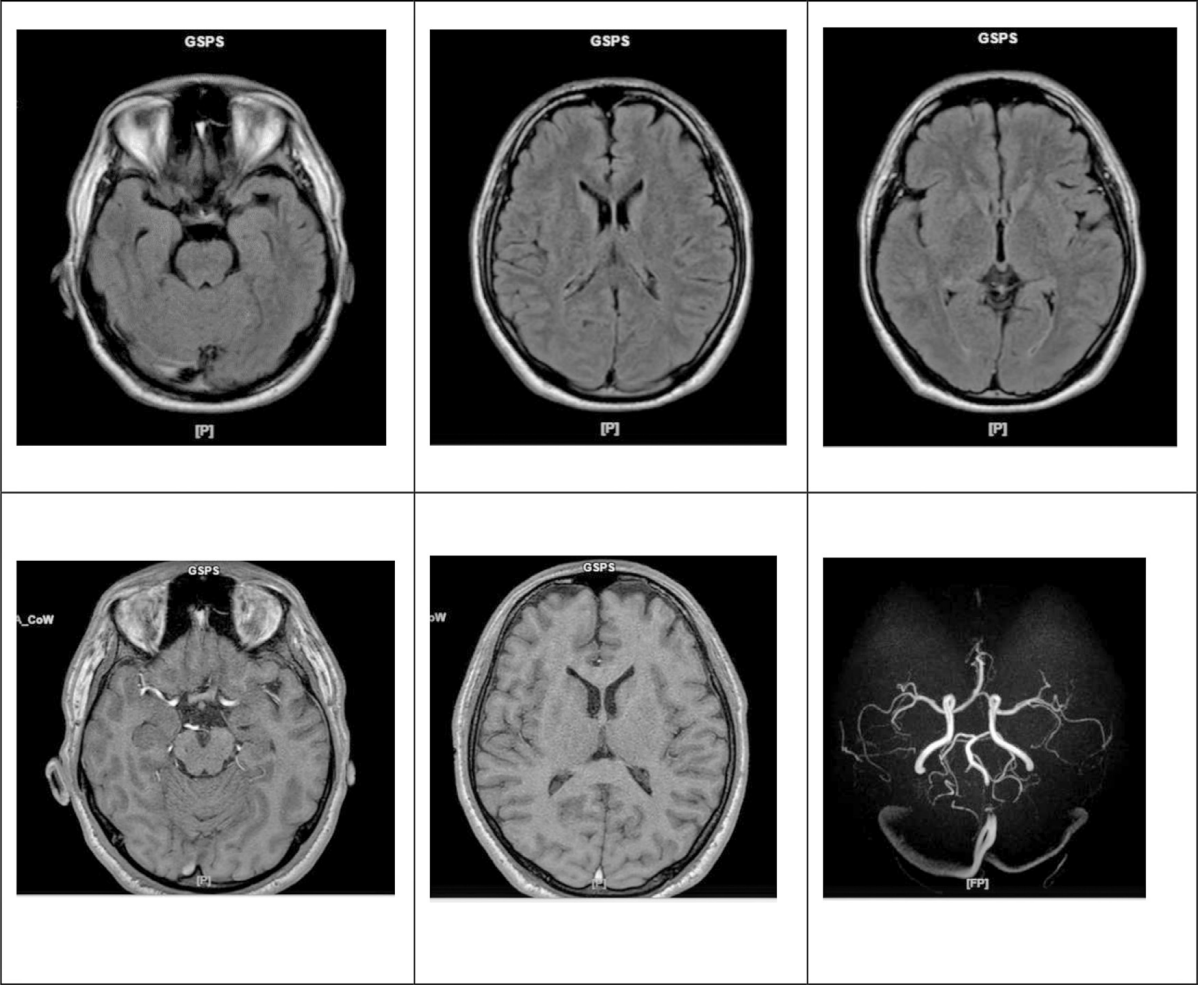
Result of noncontrast brain MRI.
